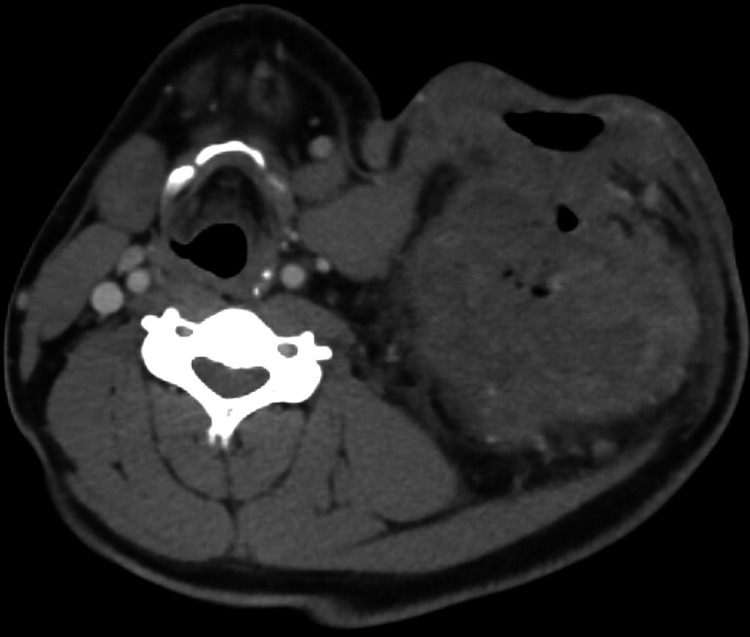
Cross-sectional image of soft tissue neck obtained using CT scan with contrast revealed that there was a large, complex, ulcerated mass in the left neck measuring approximately 12 x 10 x 15 cm. The median margin abuts the carotid sheath and there is attenuation of the internal jugular vein. The inferior margin approaches the thoracic inlet including the great vessels. This has the appearance of a necrotic, ulcerated malignancy with associated inflammation. CT: Computed tomography
